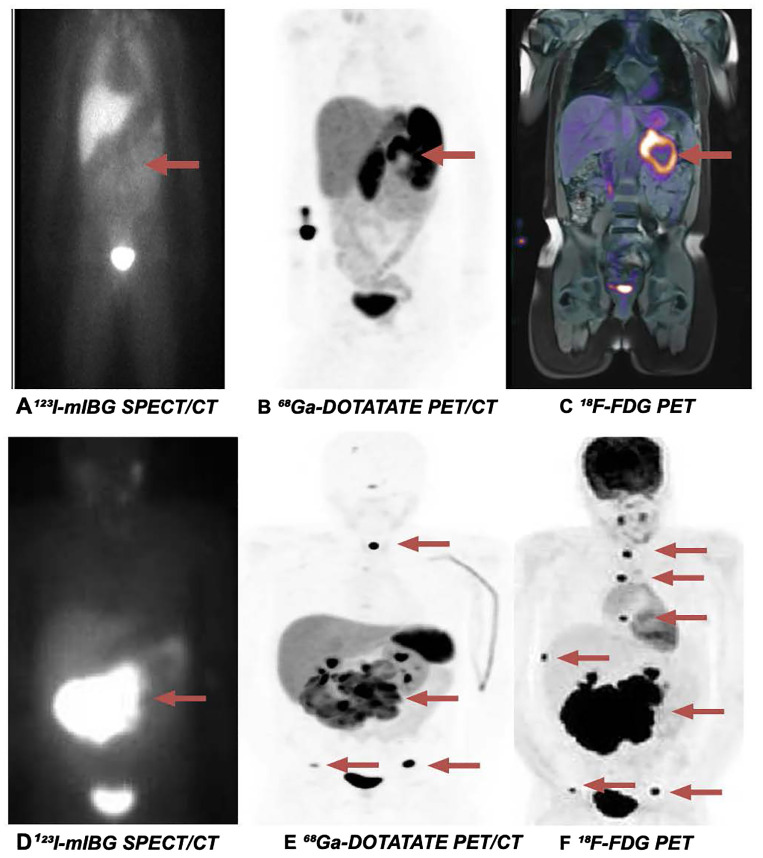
Comparison between three scanning modalities in benign and malignant disease (A–C) A patient with a benign left adrenal phaeochromocytoma, (A)^123^I-mIBG SPECT/CT shows no radiotracer uptake in the lesion, (B)
